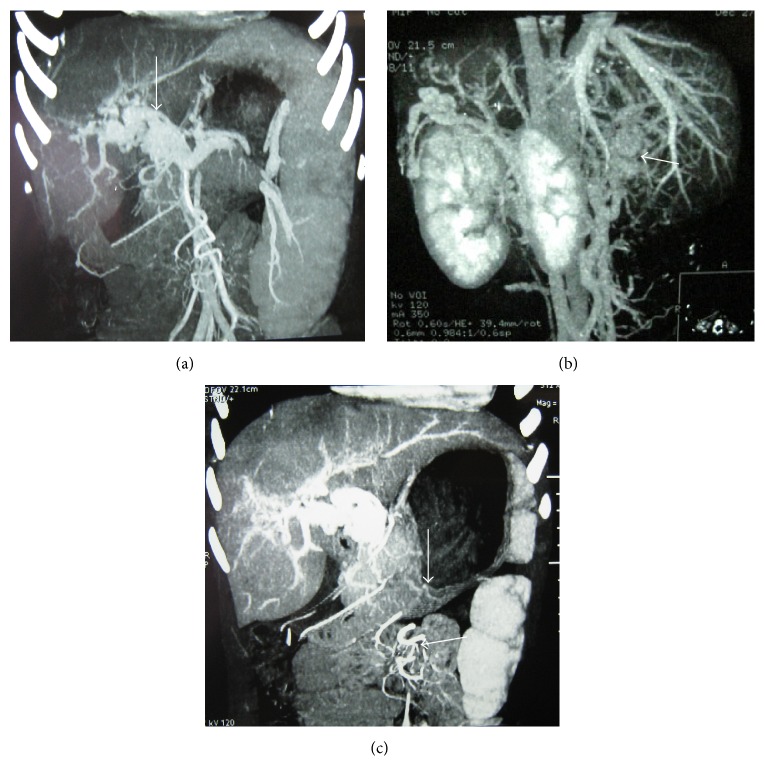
Dilated and tortuous portal vein cavernoma. Axial and coronal of sixty-four-slice CT angiography indicate (a) dilated and tortuous portal vein, (b) intrahepatic PV, and (c) collateral circulation (arrow).
